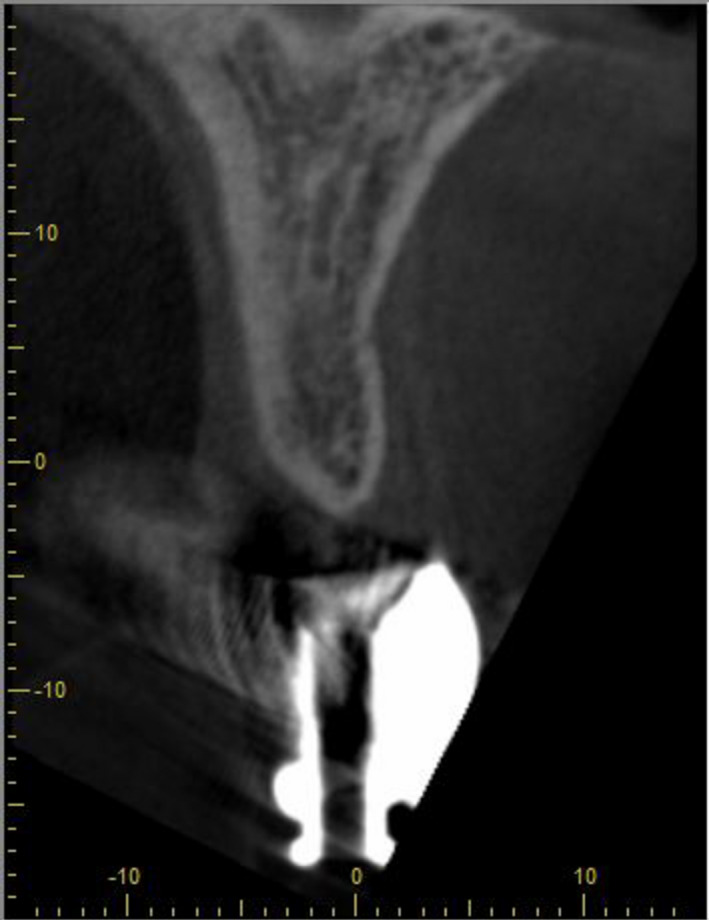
CBCT scan with surgical guide indicating horizontal bone resorption in the region of missing tooth #21 (before implantation)
